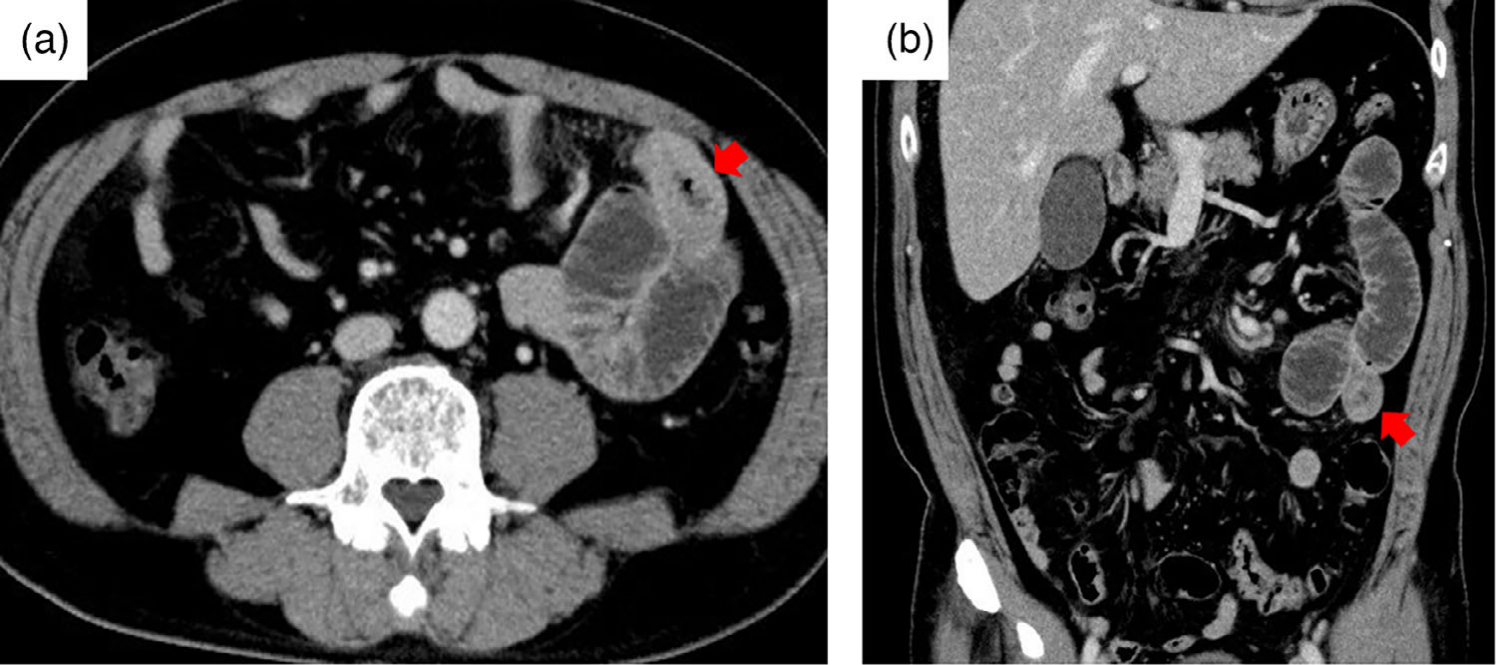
Contrast‐enhanced computed tomography on the second day of admission showed circumferential wall thickening with contrast effect in the partial segment of the small intestine (red arrow) (a, b).
